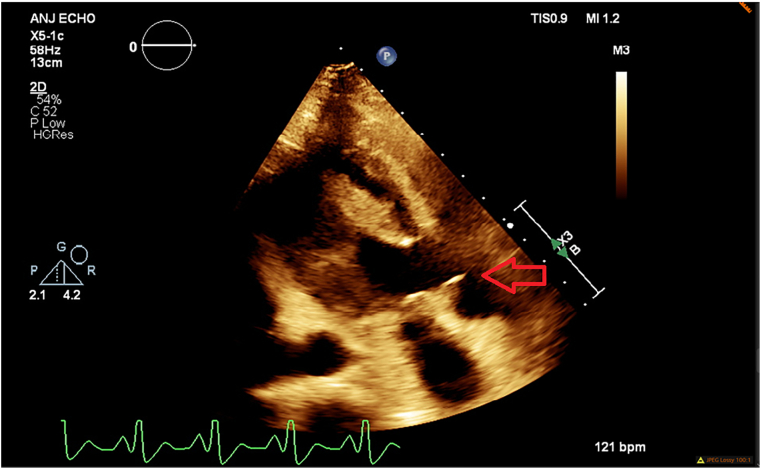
Four-chamber transthoracic echocardiography showing evidence of aortic valve nodules.
